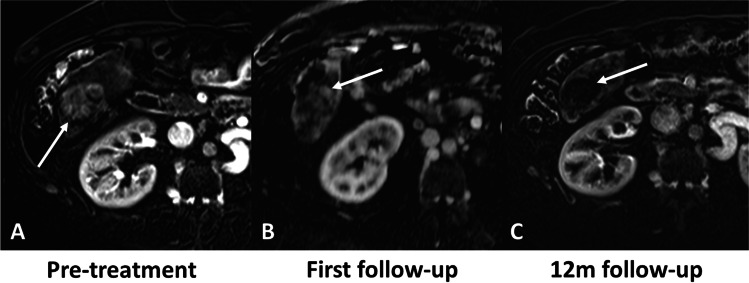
61-year-old male with HCV cirrhosis and right hepatic lobe HCC treated with radiation segmentectomy. MRI obtained during the arterial phase. Pre-treatment images show a hypervascular lesion in the right liver lobe (arrow in A). 1^st^ follow-up MRI show one hypervascular nodule within the tumor (arrow in B), and was classified as partial response according to mRECIST, LR-TR equivocal (reader 2) or LR-TR viable (readers 1 and 3) according to LI-RADS TRA and 80–90% necrotic according to subtraction by the 3 readers. The treatment response at the 12-month follow-up was categorized as complete response according to mRECIST (arrow in C)
